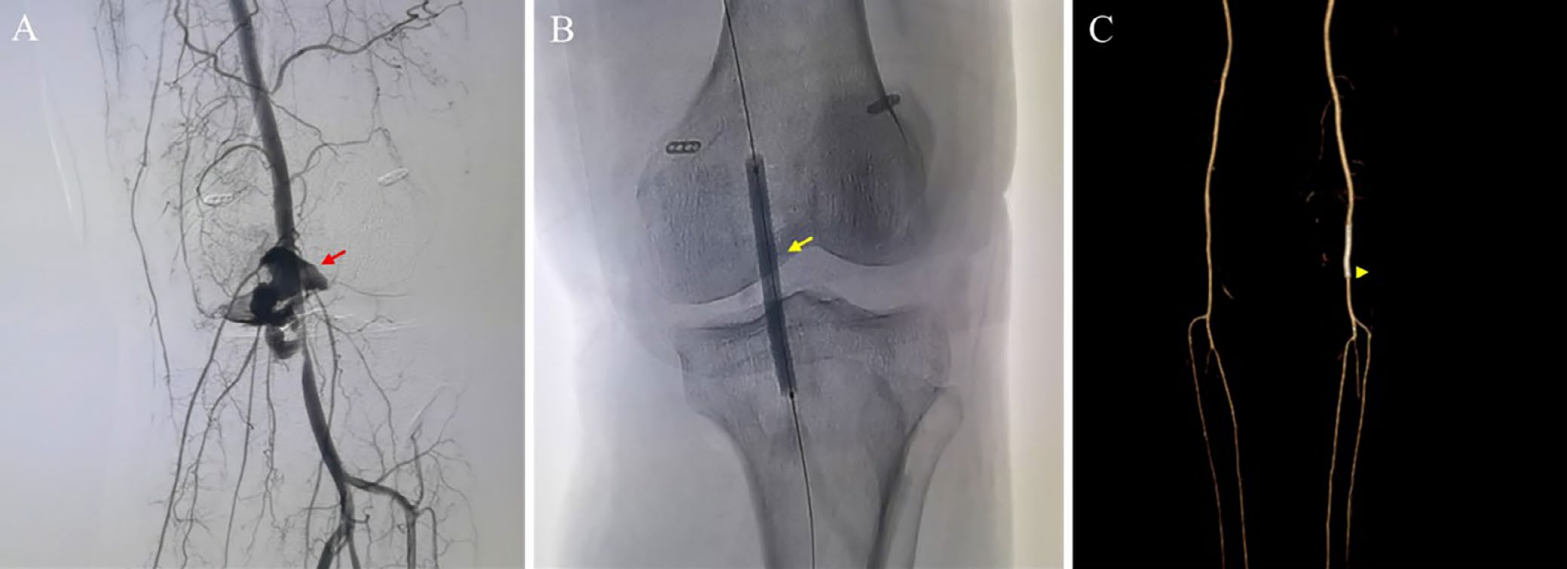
Imaging results of the patient. (A) DSA showed a ruptured popliteal artery pseudoaneurysm (red arrow). (B) A Viabahn Stent Graft was deployed and ballooned at the broken ends of the popliteal artery. (C) Postoperative CTA showed the patency of blood flow in the left popliteal artery (yellow arrow).
